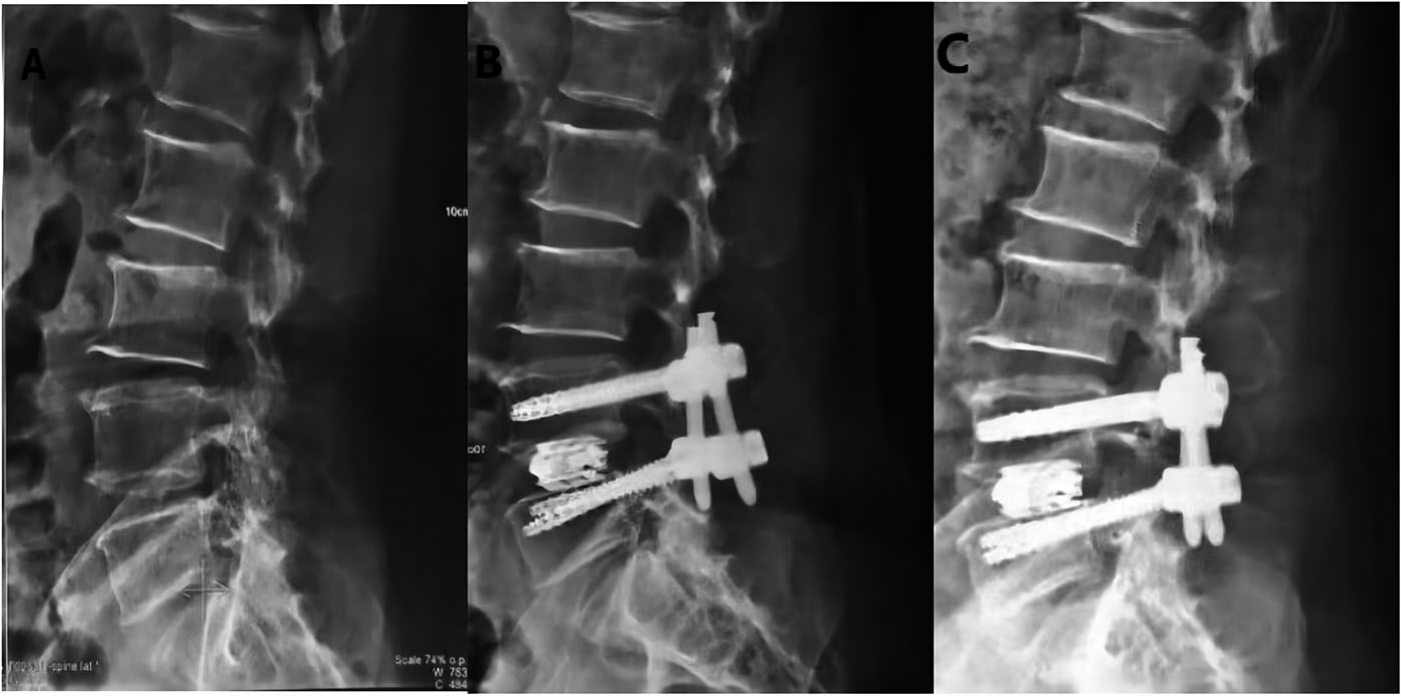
Design of EXP-IFC for anterior and Lateral display of Lumbar vertebrae after MIS-TLIF. (A) Preoperative lateral radiograph. (B) Postoperative lateral radiograph (1 week); (C) Postoperative lateral radiograph (1 year).
